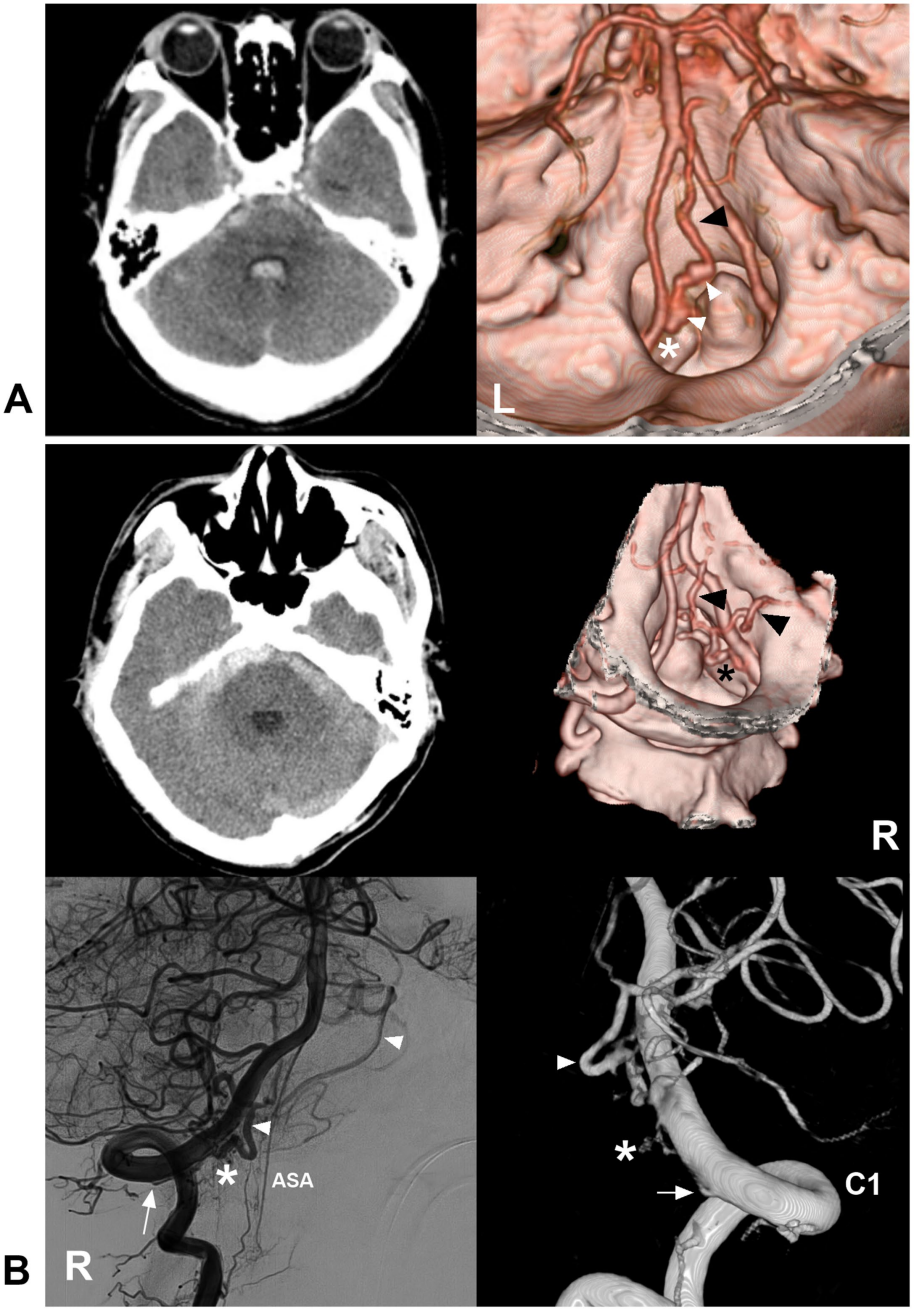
Ruptured CCJ AVF with upward drainage. (A) Left panel: CT showing subarachnoid hemorrhage and fourth ventricle hemorrhage; Right panel: CTA showing that a CCJ AVF (asterisk) had upward venous drainage (black arrowhead) with varices (white arrowheads). (B) Left upper panel: CT showing subarachnoid hemorrhage; right upper panel: CTA showing that a CCJ AVF (asterisk) had upward venous drainage (arrowheads); two-dimensional DSA (left below panel) and three-dimensional DSA (right below panel) showing a RAVF (asterisks) fed by the C1 radicular artery (arrows). The drainage went into the intracranial vein (arrowheads). AVF, arteriovenous fistula; C1, first cervical vertebrae; CCJ, craniocervical junction; CT, computed tomography; CTA, CT angiography; DSA, digital subtracted angiography; RAVF, radicular AVF.
